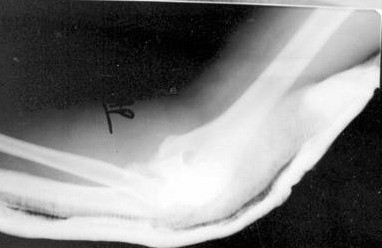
Lateral radiographs of his left elbow showed the Monteggia fracture dislocation.
